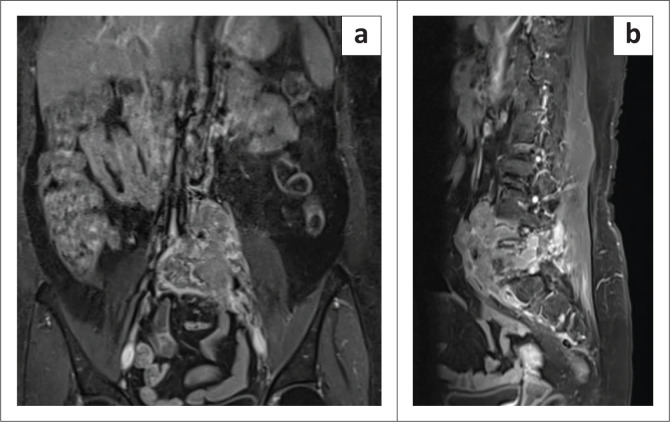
MRI coronal and sagittal post-contrast T1 Fat Saturated sequence (T1FS) images. The lesion shows marked post-contrast enhancement and is seen encasing the aortic bifurcation and left common iliac vessels (a). Sagittal image showing the cranio-caudal extension of the lesion from the anterior aspect of the inferior endplate of the L3 vertebra to the anterior aspect of the S1 vertebral body (b).
